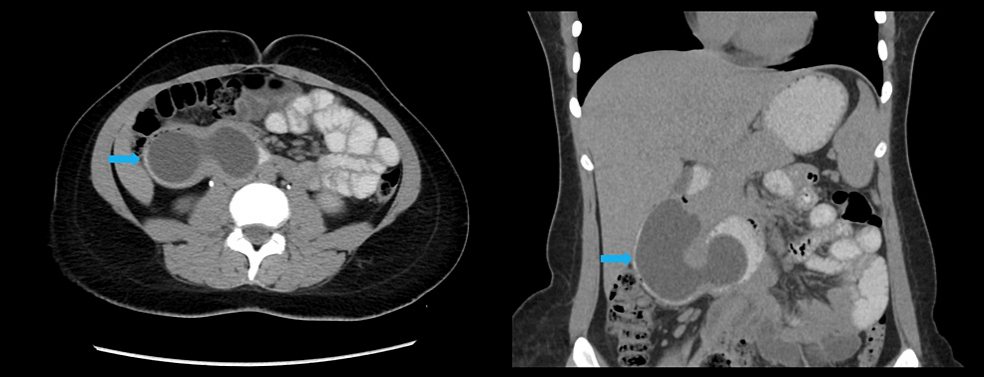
Axial (left) and coronal (right) post-oral contrast images of the CT abdomen showing an 8.3 × 4.0 cm tubular cystic lesion (blue arrows) in the second part of the duodenum The change in the shape of the tubular structure is evident across three phases: portovenous, post-oral contrast, and lateral decubitus (Figures 1-3). These findings suggest a large intraluminal DD (blue arrows) in the second and third parts of the duodenum, without signs of cholecystitis, diverticulitis, or other complications. DD, duodenal diverticulosis
